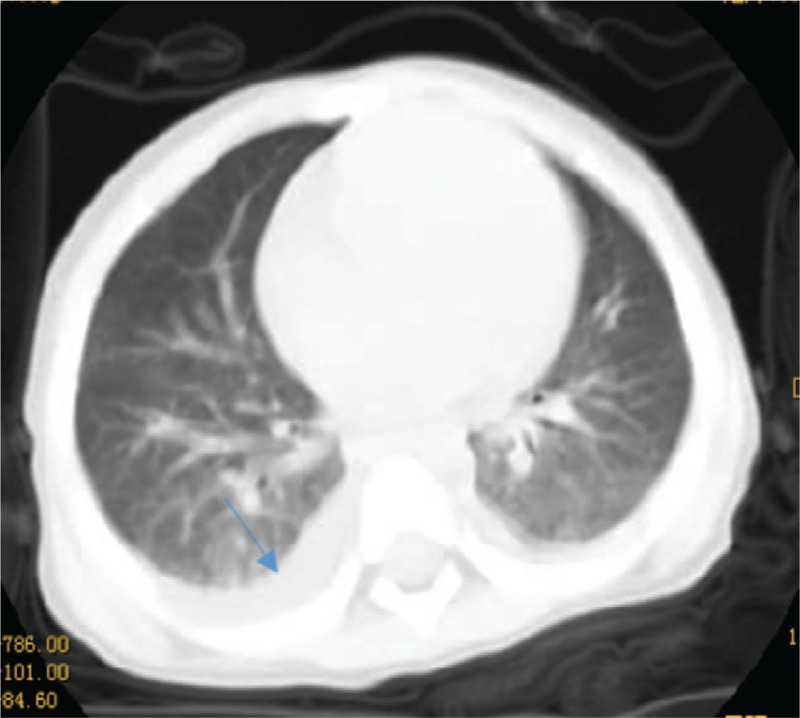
The CT of the chest showed blurred patches and streaked shadows in the lower regions of the lungs with small bilateral pleural effusions.
